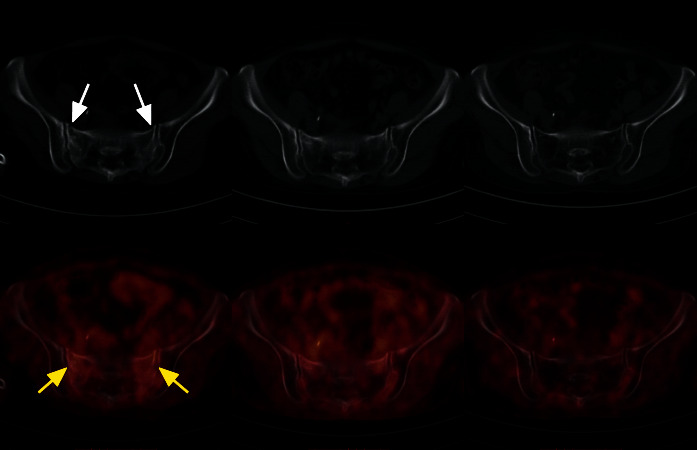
A 47-year-old woman who underwent pelvic radiotherapy for cervical cancer developed hip pain at 21 months after radiotherapy. Diffuse, mild, linear FDG uptake (left SUVmax, 3.0; right SUVmax, 2.6) parallel to the sacroiliac joint in the bilateral sacral ala (yellow arrow) was found in the fused axial image, while the low-dose CT image showed high density and a very small right anterior sacral fracture line (white arrow). Follow-up FDG PET/CT at 12 and 24 months after diagnosis of SIF. The bilateral sacral ala essentially returned to normal.
